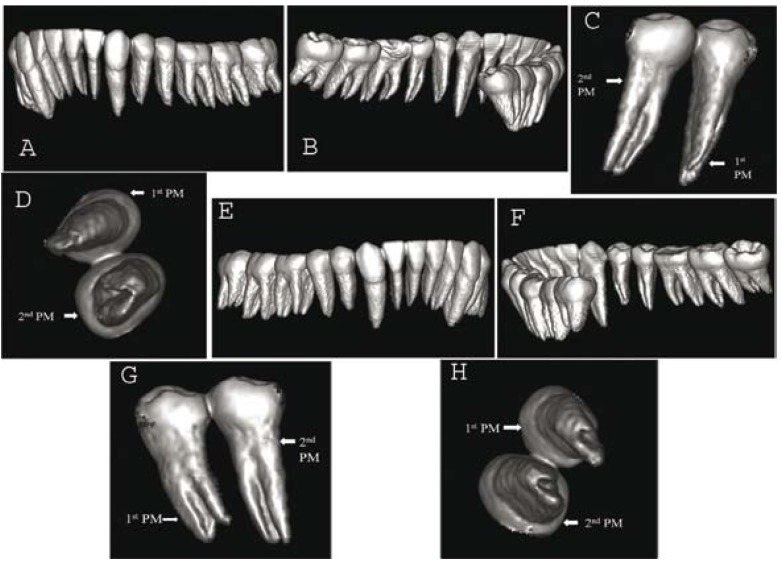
Spiral CT images; A) Three-dimensional reconstruction of the mandible (left posterior buccal view); B) Three-dimensional reconstruction of the mandible (left posterior lingual view); C) Three-dimensional reconstruction of tooth #35 and #3421 (lingual view); D) Three-dimensional reconstruction of tooth #35 and #34 (inferior view); E) Three-dimensional reconstruction of the mandible (right posterior buccal view); F) Three-dimensional reconstruction of the mandible (right posterior lingual view); G) Three-dimensional reconstruction of tooth #44 and #45 (lingual view); H) Three-dimensional reconstruction of tooth #44 and #45 (inferior view).
